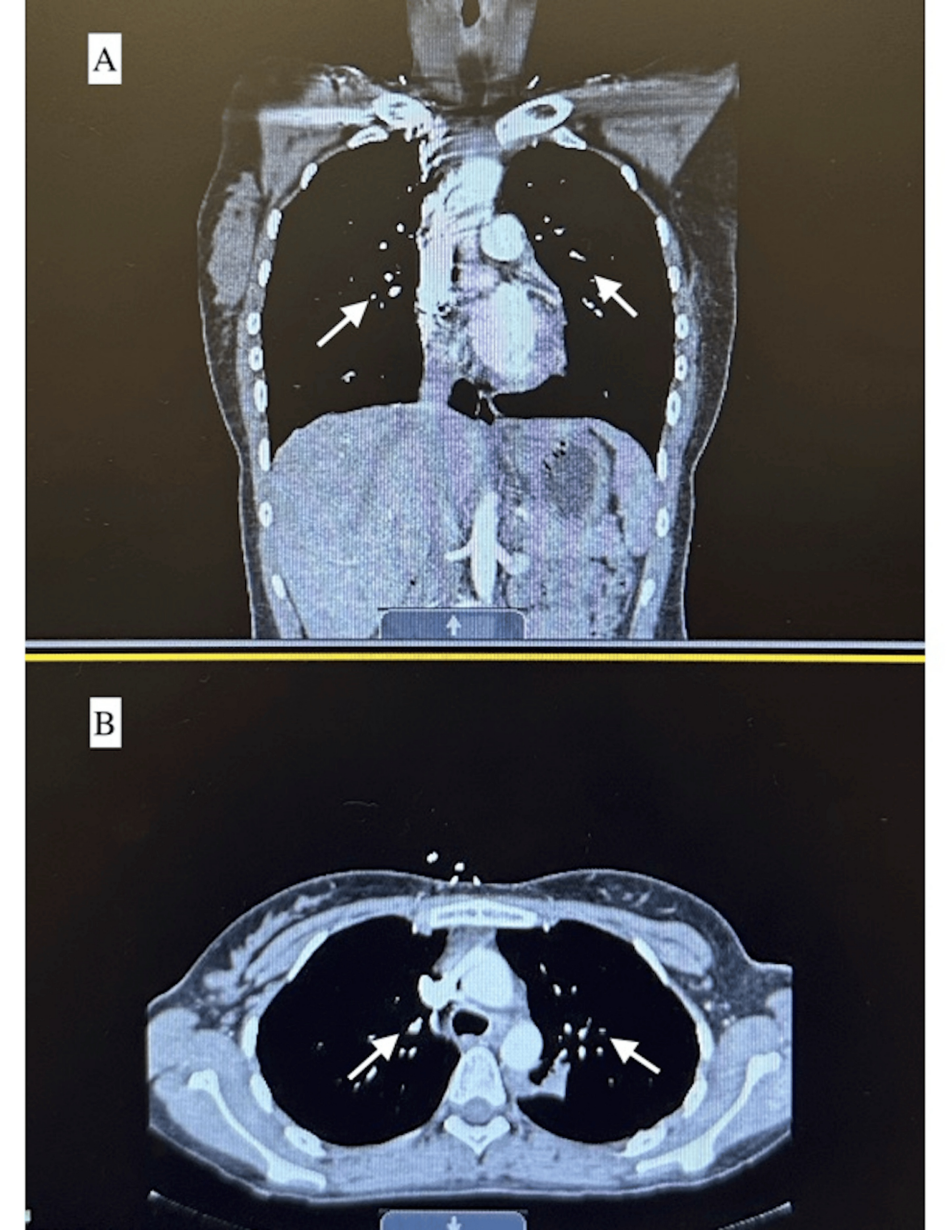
CT of the chest A) Coronal CT scan of the chest that shows mass-like infiltrates within the left and right lung cavities (white arrows); B) Axial CT scan of the chest that shows mass-like infiltrates within the left and right lung cavities (white arrows)
